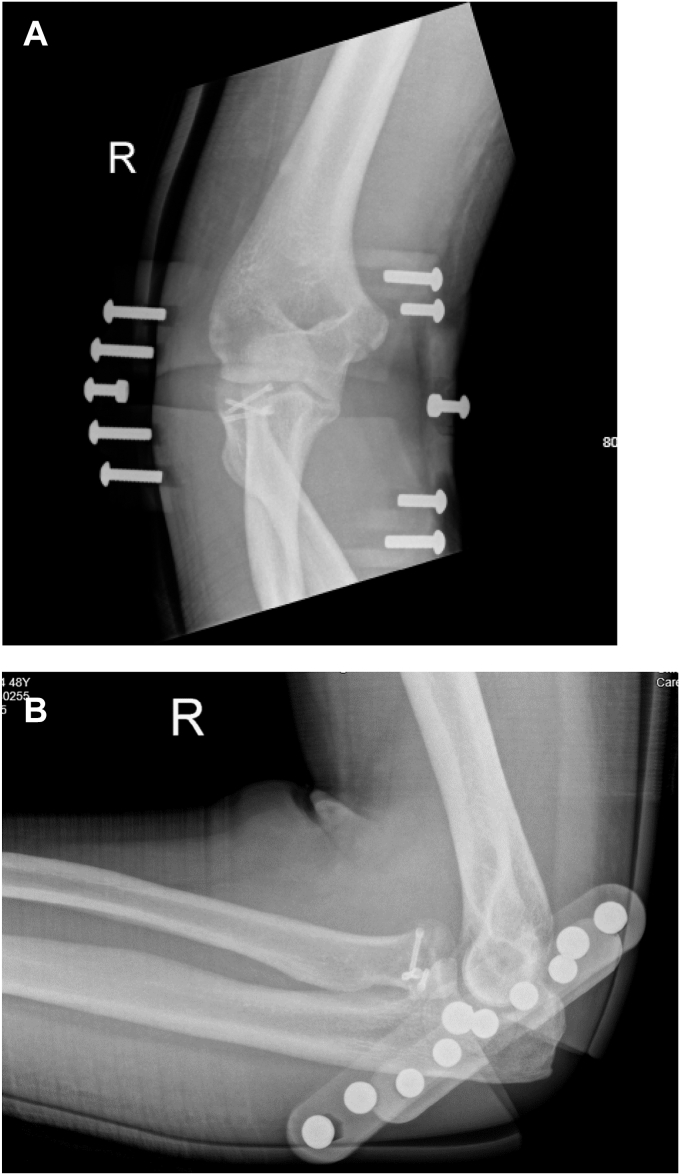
X-rays in splint. (A) Anteroposterior view. (B) Lateral view.
